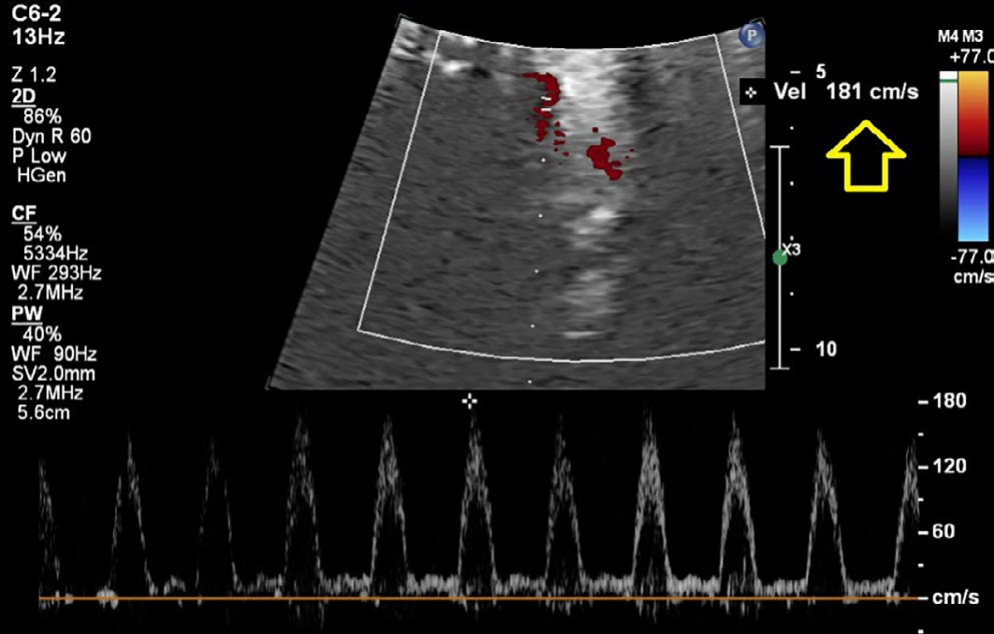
Spectral Doppler interrogation at the stenotic segment of the ductus arteriosus reveals elevated peak flow velocity, consistent with hemodynamic narrowing.
